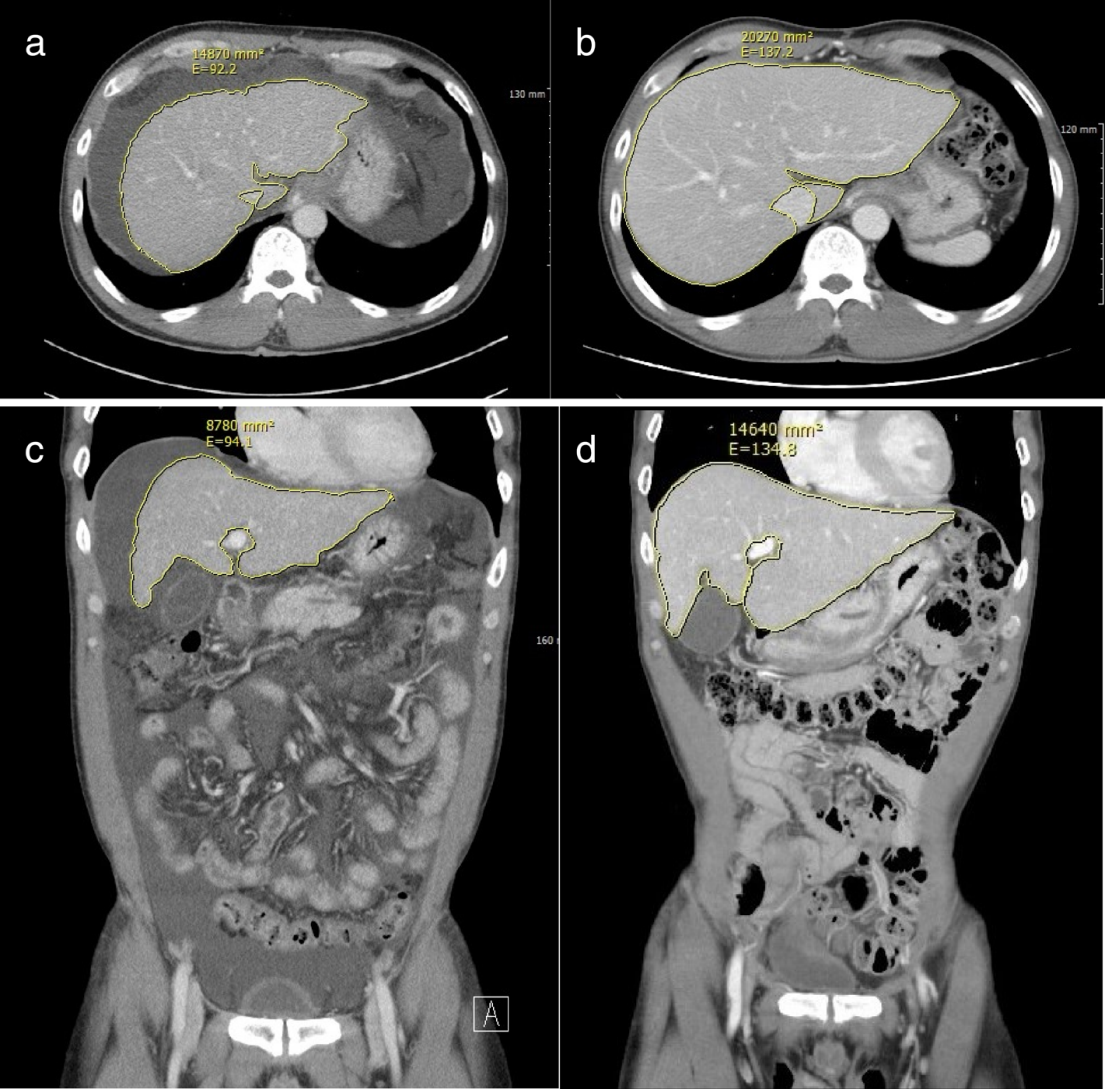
Computed tomography (CT) images taken (a) before mesenchymal stem cell infusion, showing ascites and a liver volume of 14 870 mm^2^, and (b) at 80 weeks after infusion, showing reduced ascites and a liver volume of 20.270 mm^2^. (c) Coronal CT view before treatment showing a huge amount of ascites and a liver volume of 8780 mm^2^. (d) Coronal CT view at 80 weeks after treatment showing improved ascites and a liver volume of 14.640 mm^2^. Images with largest liver volumes were chosen for the CT images.
